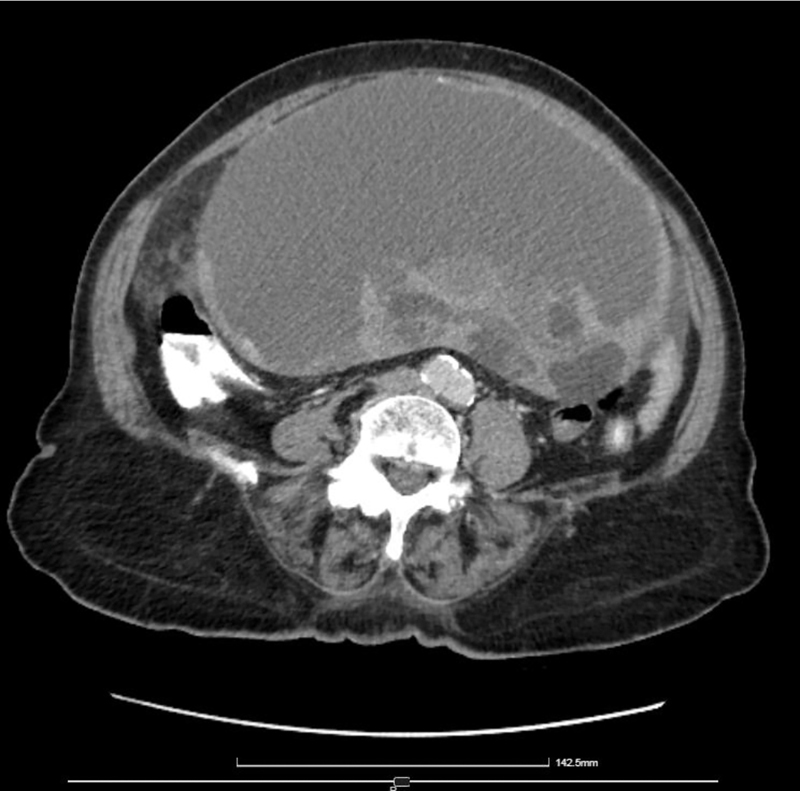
Computed tomography (CT) of the mass.
